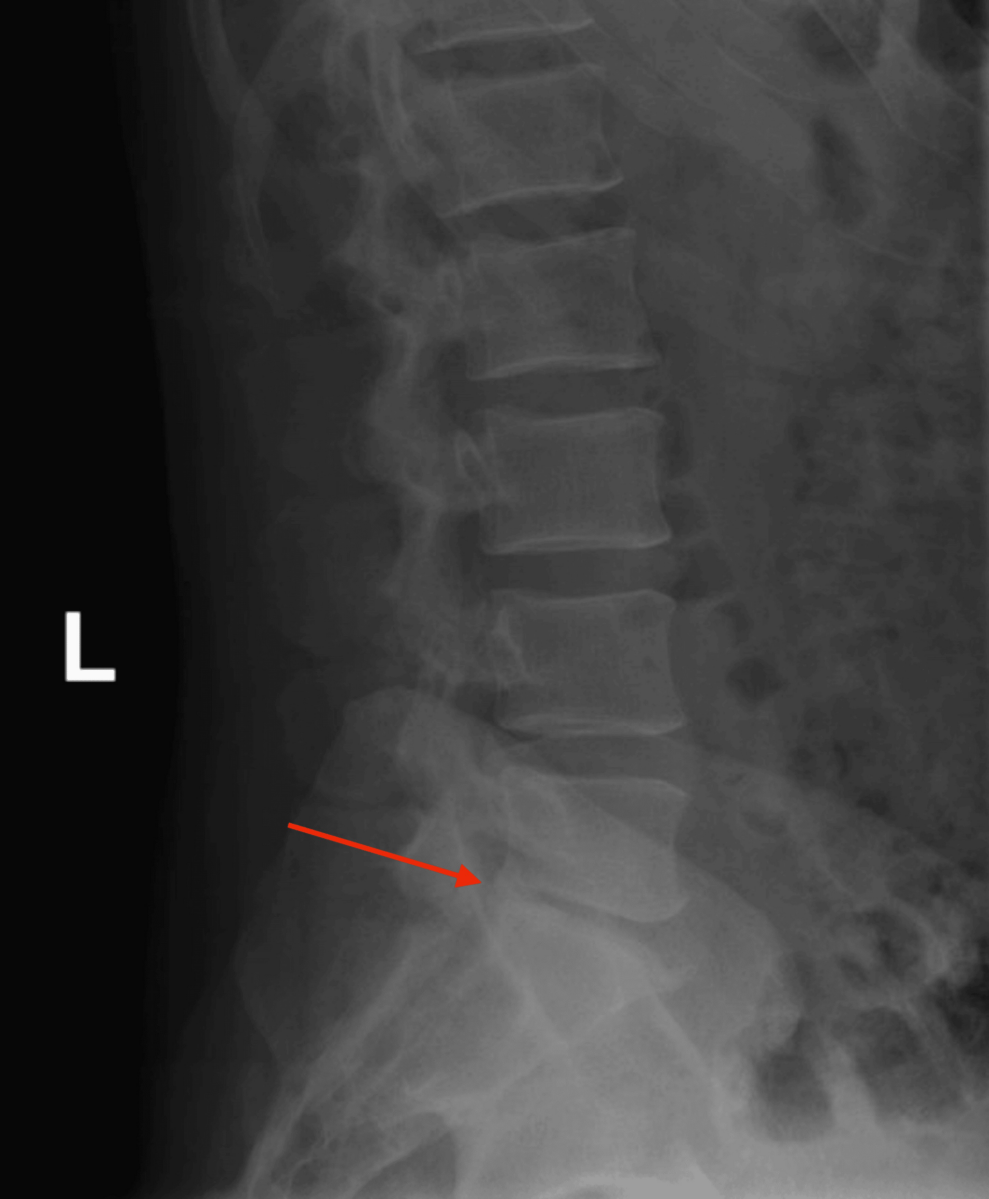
Lateral lumbar spine radiograph demonstrating severe degenerative changes at the L5–S1 disc space (red arrow), including disc space narrowing and endplate sclerosis.
